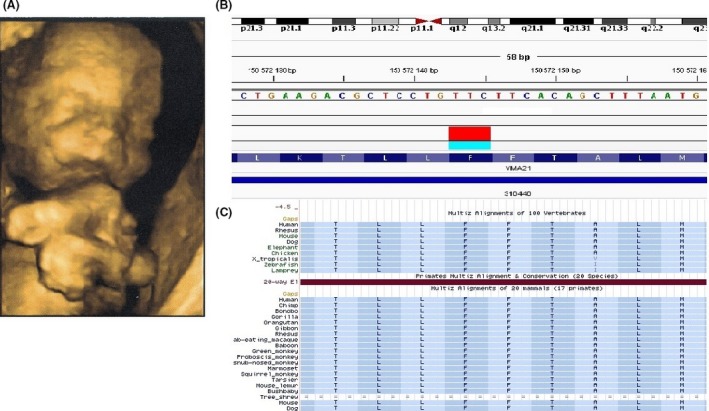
(A) Sample picture of level II ultrasound of male fetus. (B) IGV view of the *VMA21* gene c.94_96delTTC (p.Phe32del) nonframeshift deletion mutation. (C) UCSC Genome Browser alignments of the *VMA21* gene mutation region.
